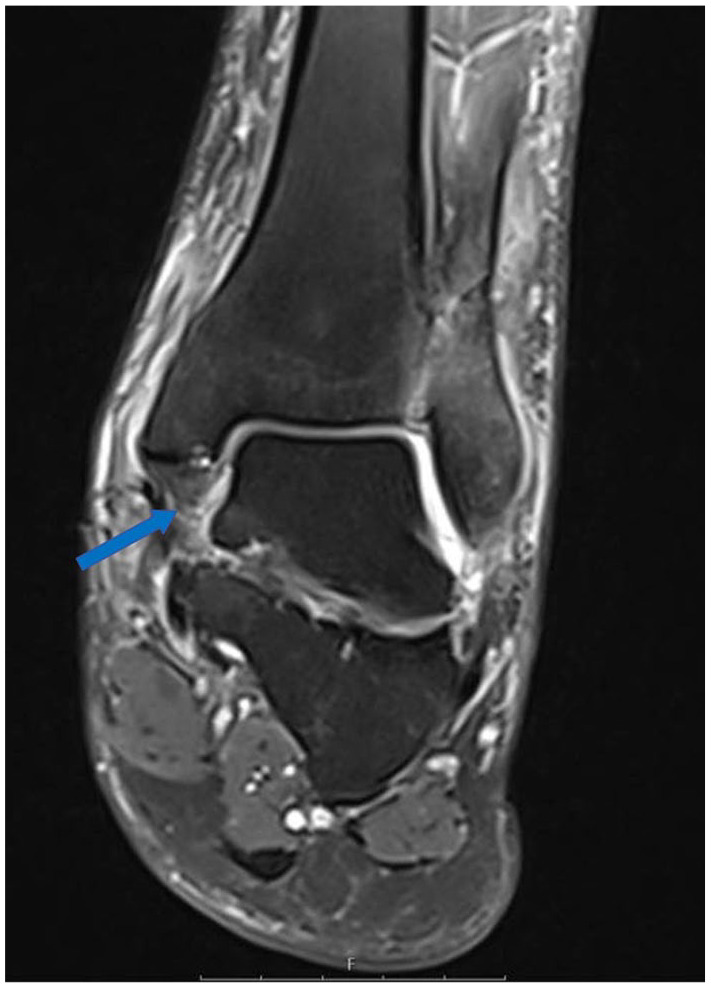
Magnetic resonance image (coronal, proton density-weighted fast spin echo) showing a total rupture of the dPTTL (arrow). dPTTL, deep posterior tibiotalar ligament.
